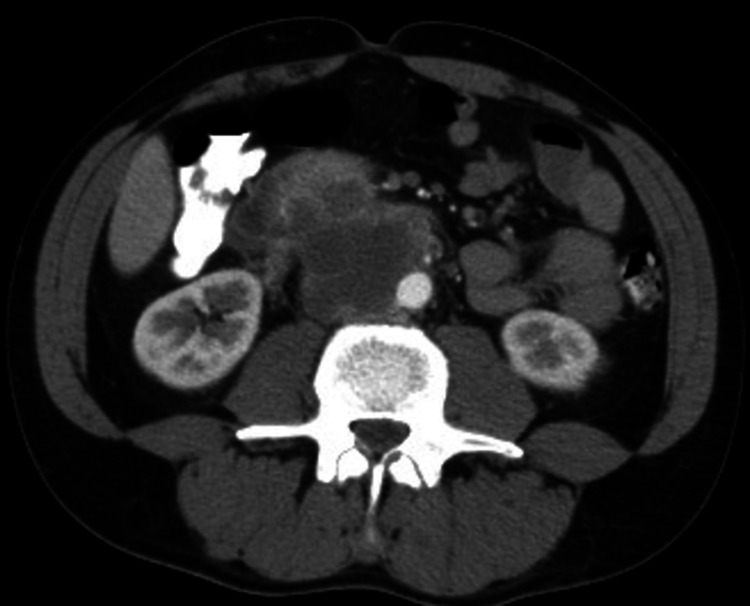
Contrast-enhanced CT scan of the abdomen revealing a large retroperitoneal mass
